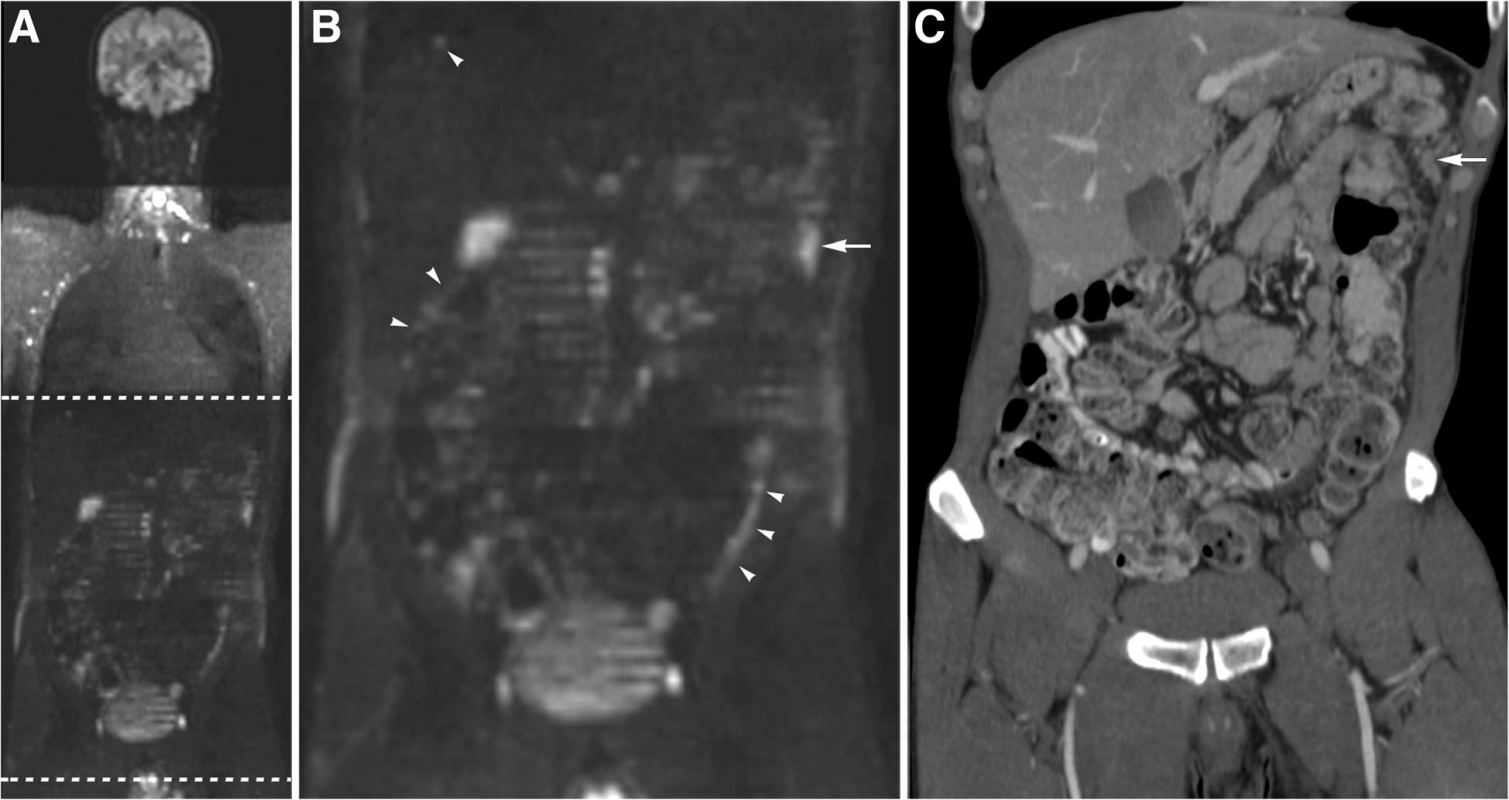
A 51 year old male patient with cancer at the rectosigmoid junction and clinical suspicion of peritoneal metastases underwent a WB/DWI-MRI (a/b) in addition to a CT scan (c) to evaluate operability. WB/DWI-MRI showed extensive peritoneal cancer spread, partially shown by the white arrowheads in the zoomed-in image (b) of the coronal b1000 DWI (a), with an estimated PCI of 26. The tumour deposit at the splenic flexure of the colon (arrow in b) was also recognized on CT, shown by the white arrow on the coronal CT image (c), but the other lesions were not seen on CT. Disease load was clearly underestimated with an estimated PCI of 8. At explorative laparotomy extensive peritoneal disease was confirmed (PCI 33) and the patient received optimal palliative care
